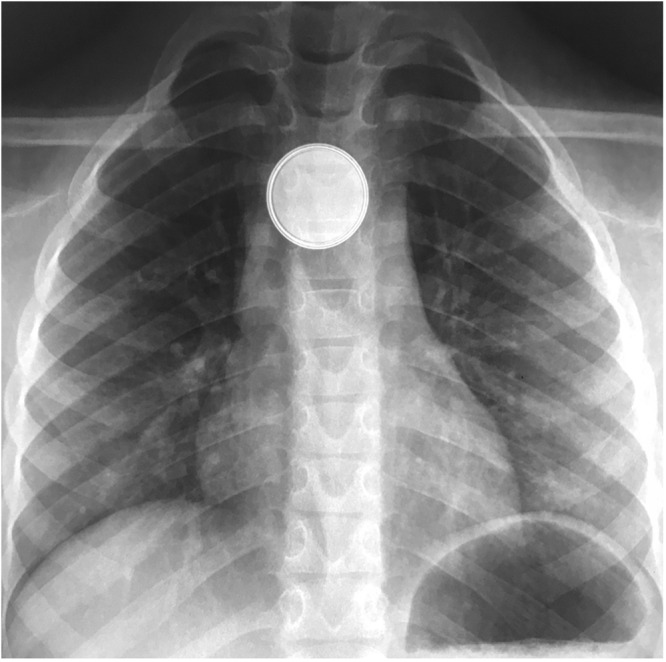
Halo sign (double ring sign) on anterior–posterior view chest x-ray.^20^
